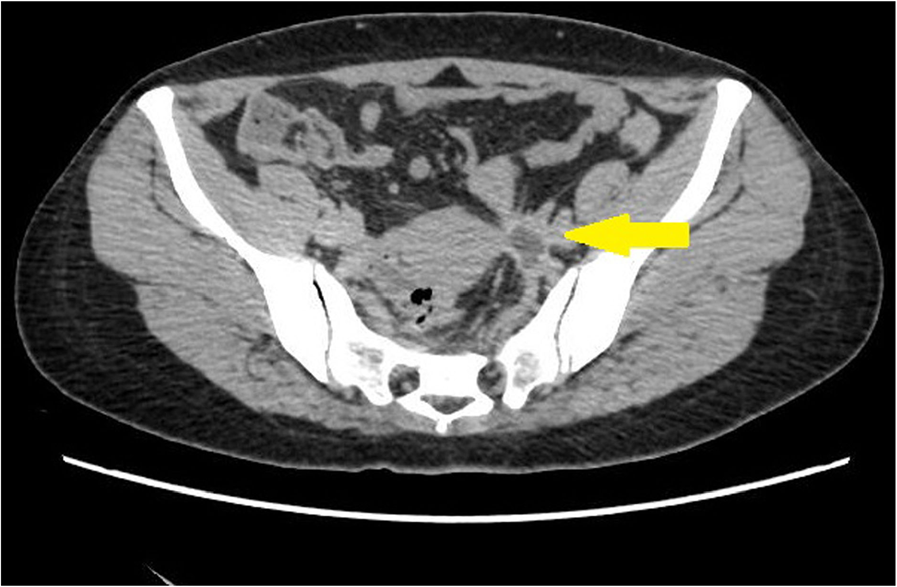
Pelvic CT scan showed a thick-walled cystic mass (arrow). The left ureter could not be clearly identified
